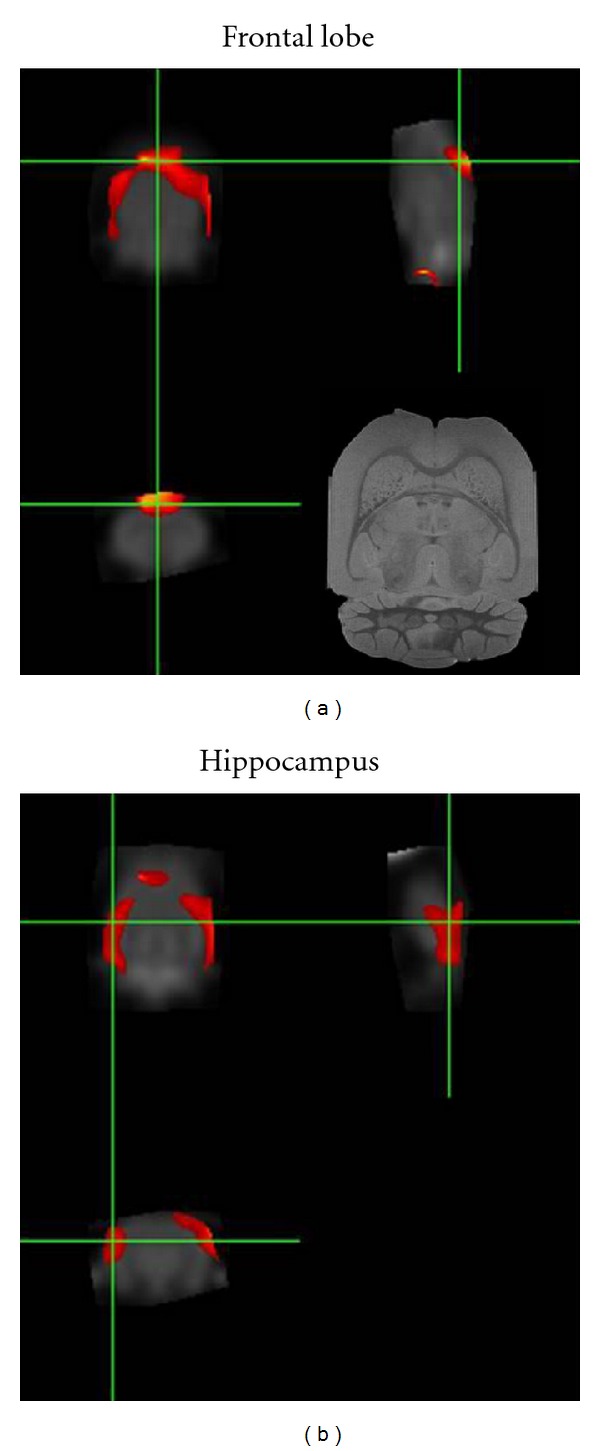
Brain regions where regional FDG uptakes in Squid-PS were significantly higher than in control (hippocampus and frontal lobe).
